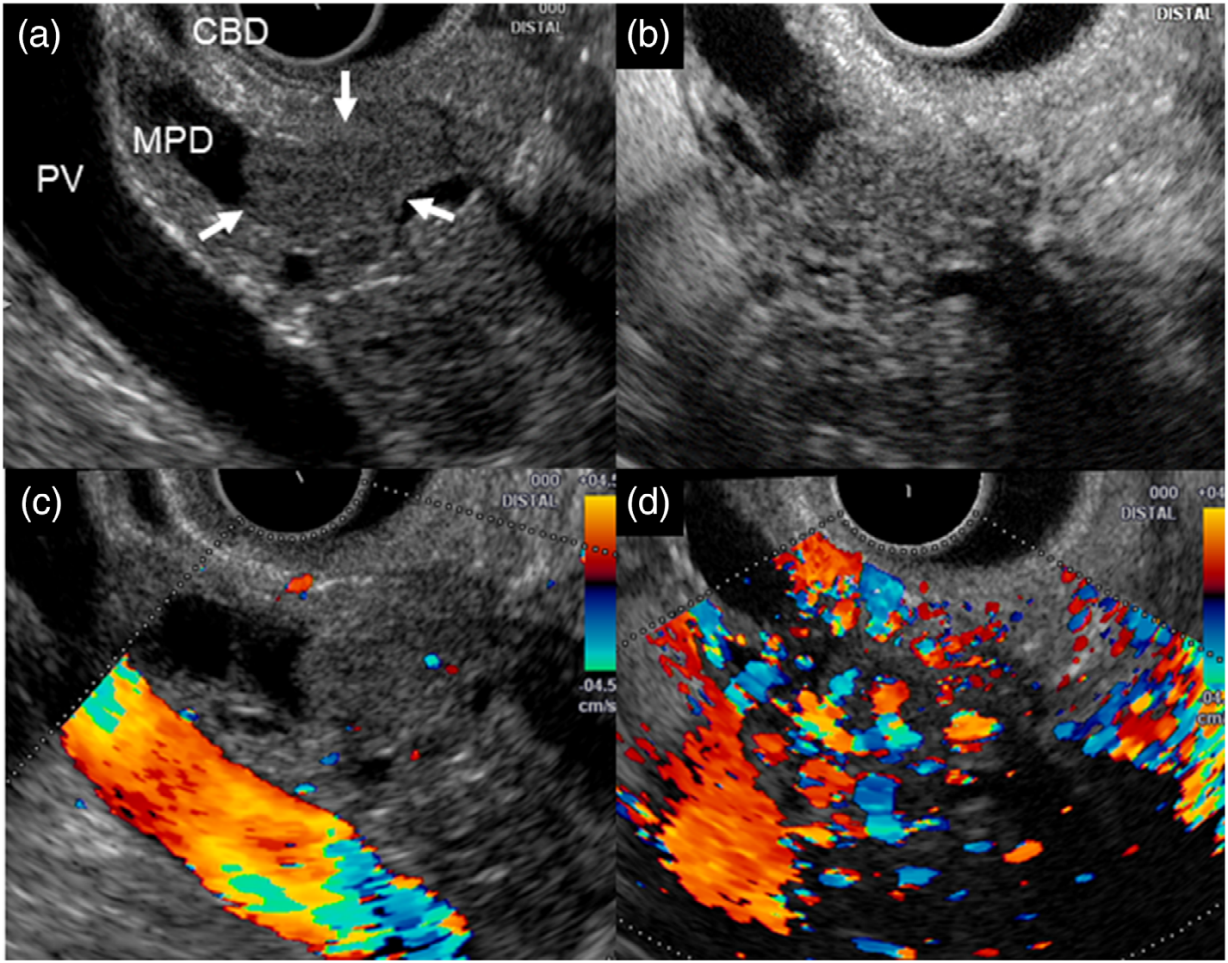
(a) Endoscopic ultrasonography (EUS) showing an isoechoic mass of 12 mm in size (arrows) in the main pancreatic duct of the head of the pancreas, and the caudal duct was markedly dilated. The common bile duct was located close to the mass, but there was no dilatation. (b) Contrast‐enhanced harmonic EUS using Sonazoid showing rapid enhancement at an early stage (20 s). (c, d) Colour doppler mode imaging (c) before Sonazoid injection, (d) 60 s after Sonazoid injection showing abundant blood flow signals in the mass after Sonazoid injection. PV: portal vein, CBD: common bile duct, MPD: main pancreatic duct
